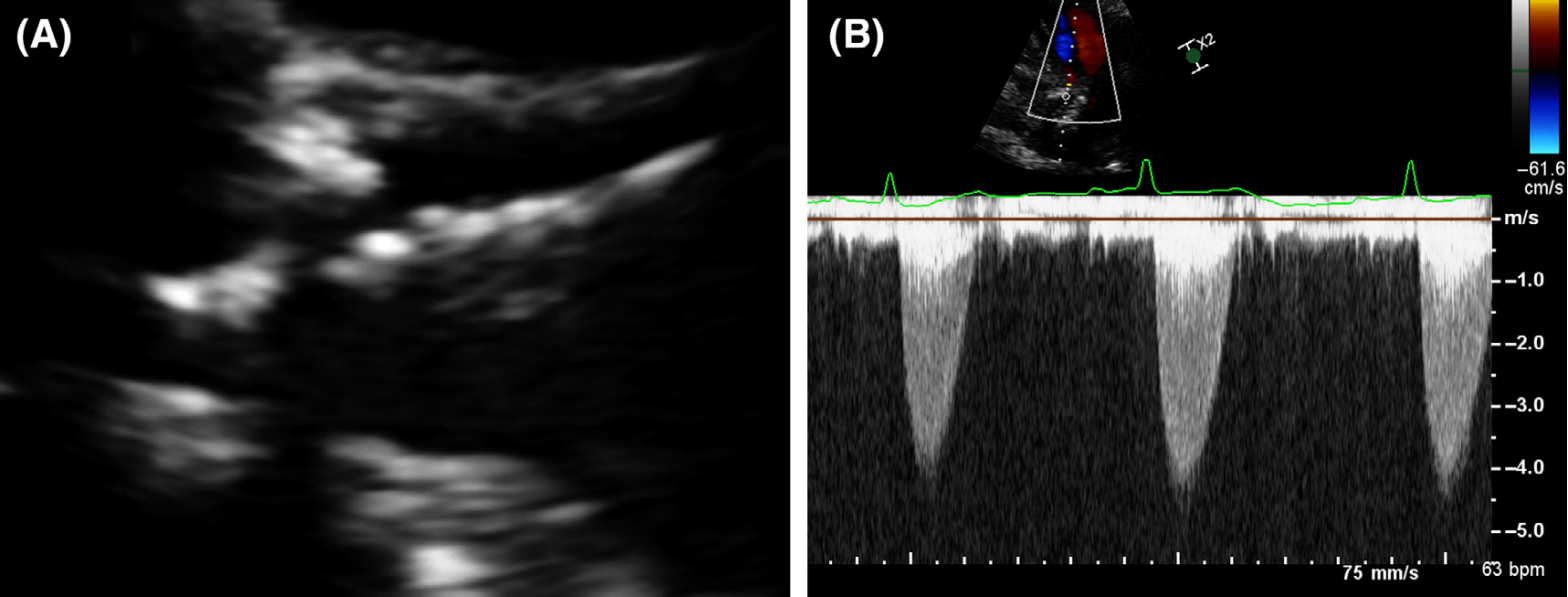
Post‐TAVR echocardiographic images and gradients. (A) 2D long‐access view transthoracic echocardiographic of bioprosthesis shows underexpansion of valve. (B) Doppler gradient was significant at mean/peak gradients of 42/72 mmHg.
